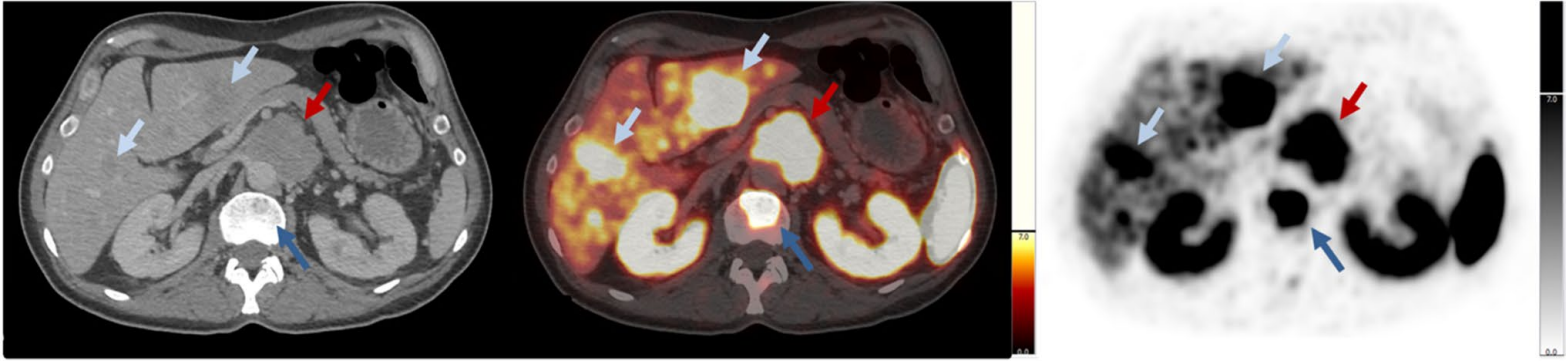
[^18^F]SiTATE-PET/CT showing distinct tracer uptake in a 51-year-old male patient with untreated sporadic paraaortic paraganglioma and metastatic disease. Displayed are axial CT (soft tissue window), axial PET/CT (soft tissue window), and axial PET images. Red arrow: primary paraaortic lesion; light blue arrows: liver metastases; dark blue arrow: osseous metastasis
